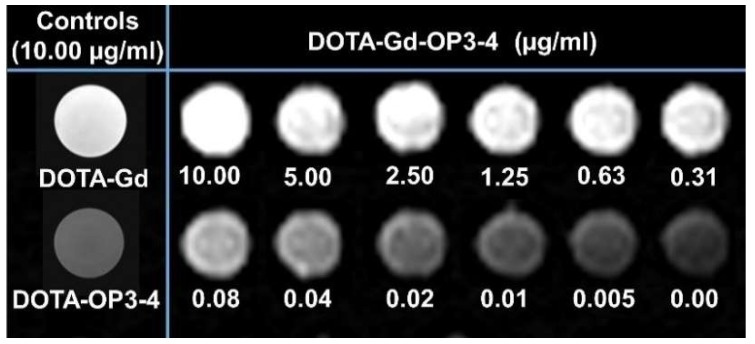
T1 weighted MRI scan of DOTA-Gd-OP3-4 showing a concentration-dependent signal intensity. The peptides, pre-dissolved in DMSO, were diluted to the desired concentration in PBS buffer (1 mL) and the MRI signal acquired at the same time using the parameters: SL5, TE 8.7, and TR550. DOTA-Gd (MW 557.64 g/mol) and DOTA-Gd-OP3-4 (MW 2141.4 g/mol) were prepared at a concentration of 1 µg/ml, corresponding to 1.79 µM and 0.47 µM Gd, respectively.
